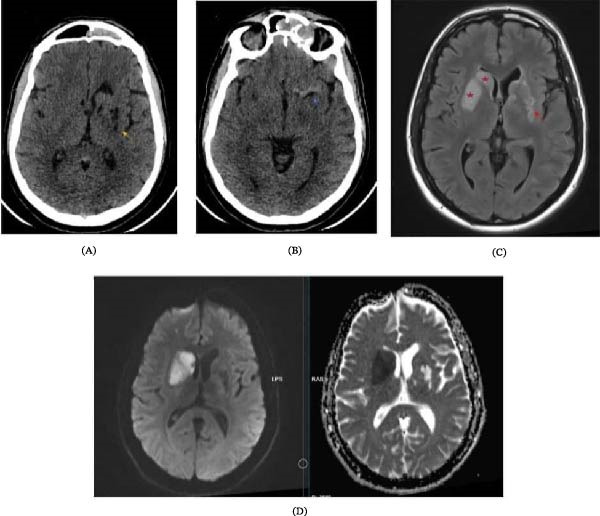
AXIAL CT and MRI images: (A and B) axial nonenhanced CT, (C) axial FLAIR MRI, and (D) DWI/ADC. Multiple hypodense areas are shown on CT (yellow arrow), representing areas of encephalomalacia with gliosis as shown on FLAIR images (red arrow). The hyperdense irregular left MCA (blue arrow). These areas are represent an old ischemic insult.
